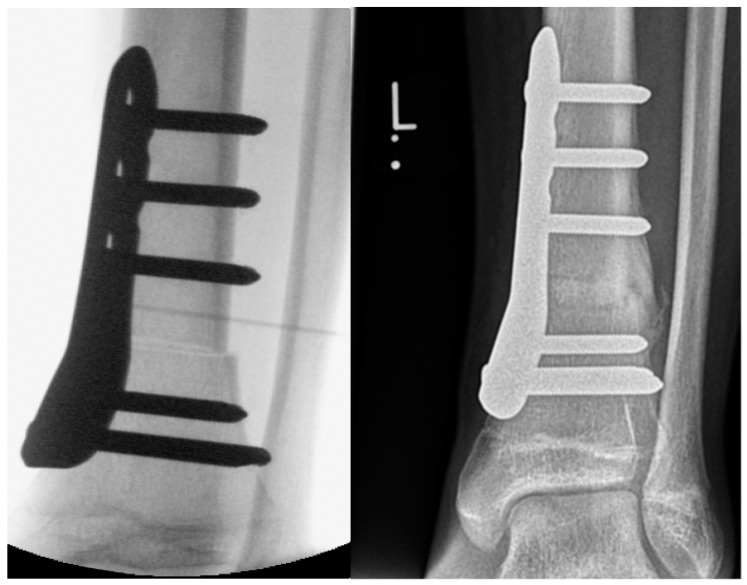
Intraoperative X-ray image and X-ray image obtained 3 months after SMO fixed with an angular stable plate in a 14-year-old male patient with increased external torsion of the tibia.
